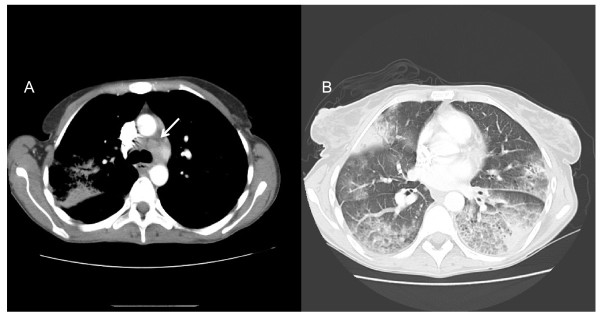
Thoracic CT Scan of case 1 at presentation. A: Mediastinal lymphadenopathies. B: Bilateral ground glass opacities and areas of consolidation.
